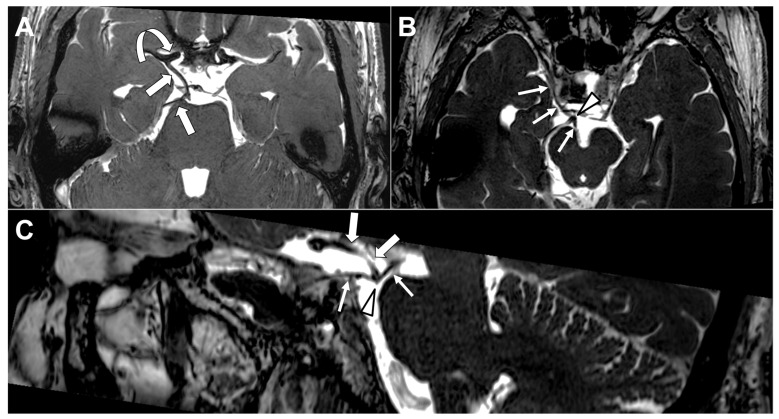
Fetal-origin posterior cerebral artery (PCA) causing neurovascular conflict with the oculomotor nerve (OMN). (A) Axial 3D high-resolution T2-weighted balanced fast field echo (BFFE) image with minimum intensity projection (MinIP) reconstruction demonstrates a fetal-origin right PCA (thick straight arrows) arising directly from the supraclinoid segment of the internal carotid artery (curved thick arrow). (B) A different MinIP reconstruction shows focal malalignment and “tenting” of the cisternal segment of the right OMN (thin arrows) at the site of vascular contact (arrowhead), consistent with compressive neurovascular conflict by the fetal PCA. (C) Sagittal MinIP reconstruction clearly depicts the angulation and inferior displacement of the cisternal OMN (thin arrows) produced by the adjacent fetal-origin PCA (thick arrows) at the site of neurovascular conflict (arrowhead). Image Credit: Dr. Nikolaos-Achilleas Arkoudis and Prof. Georgios Velonakis.
